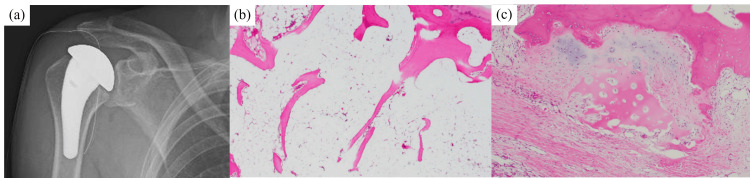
Postoperative image and pathological findings. Plain radiograph after hemiarthroplasty and osteochondral autografting (a).  Pathological findings revealed a decrease in the number of trabeculae (b) along with callus formation and tissue granulation (c). These findings led to a diagnosis of subchondral insufficiency fracture.
